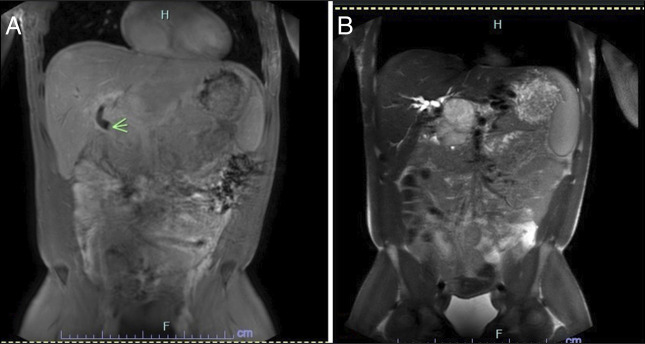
Magnetic resonance imaging of the abdomen images showing abrupt cutoff of the midcommon bile duct due to the periportal mass (green arrow).
